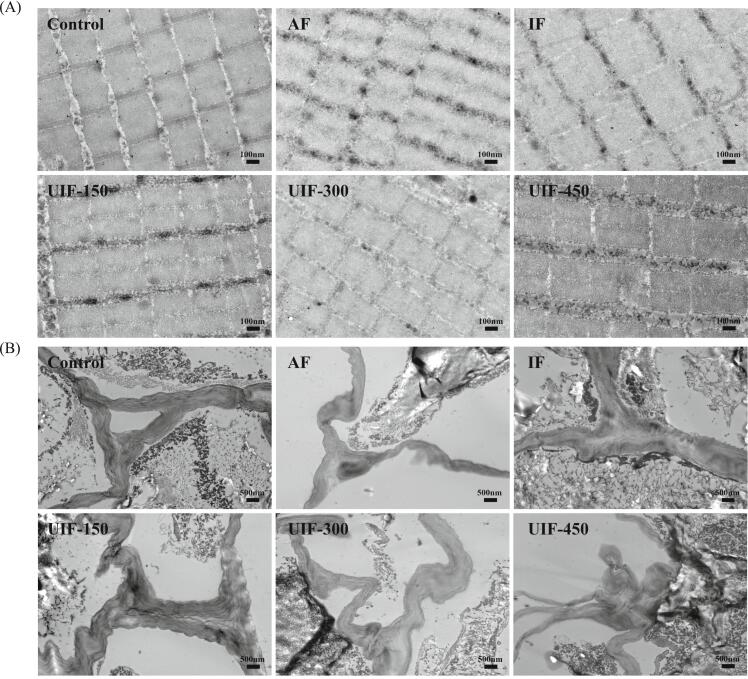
Ultrastructure of beef (A, magnification: 12000×) and potatoes (B, magnification: 3000×) in braised beef with potato dishes under different freezing treatments. AF, air freezing; IF, immersion freezing; UIF, ultrasound-assisted immersion freezing at different ultrasound powers (150, 300 and 450 W).
